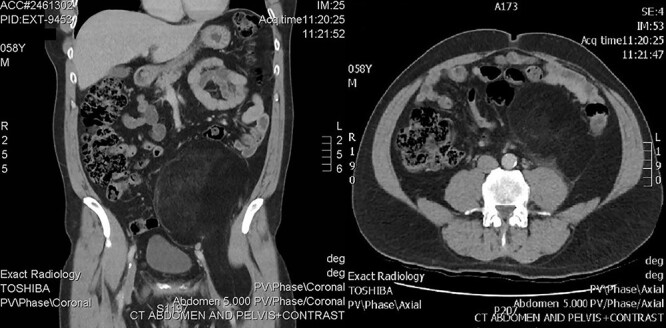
CT showing retroperitoneal mass.
